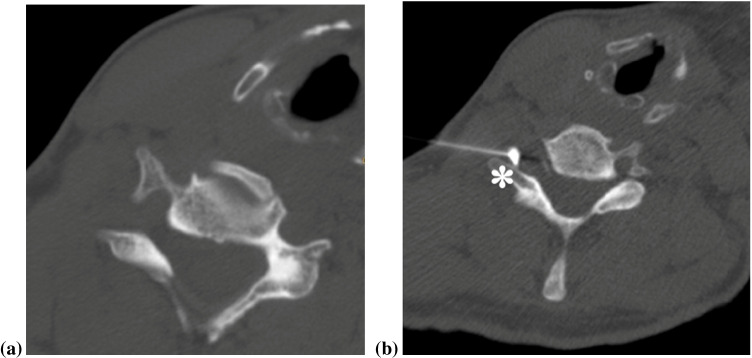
Example of the direct nerve root injection technique for the cervical spine which was abandoned in our institution more than a decade ago due to safety reasons. CT-guided left-sided direct C6 cervical nerve root block in a 43-year-old female patient. The patient is in a supine position, with the head slightly tilted to the contralateral side. After a planning CT (a), the needle was inserted from the lateral side, and the position of the needle tip next to the cervical nerve root (asterisk) was verified with a contrast injection (b)
